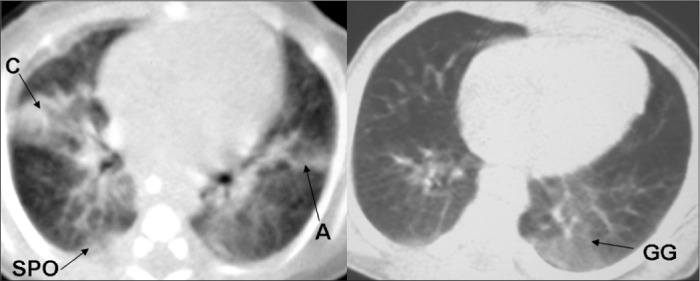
High-resolution computed tomography (HRCT) scan showing atelectasis (A), consolidation (C), subpleural opacity (SPO) and ground-glass opacity (GG).
